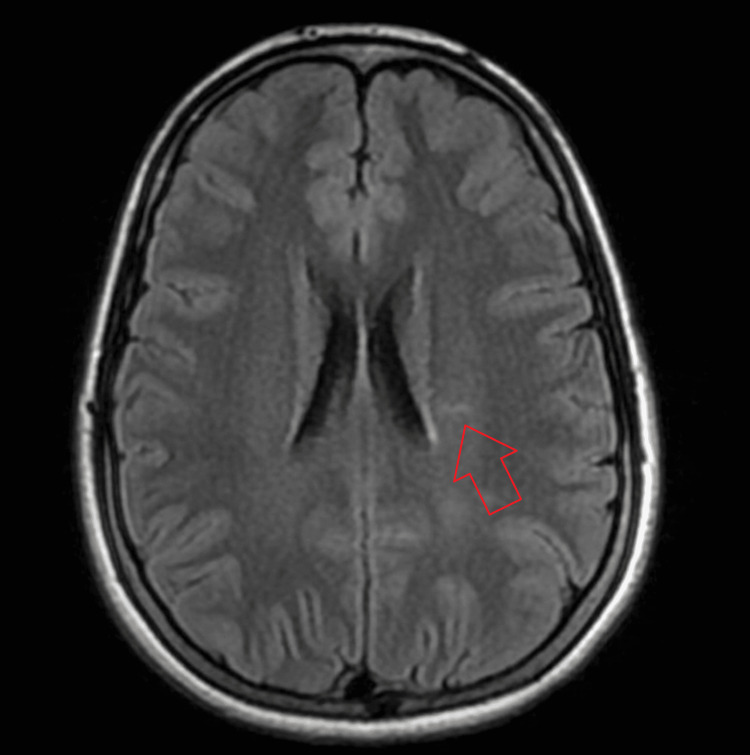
Cranial MRI findings (axial T2-FLAIR sequences) demonstrating a linear periventricular deep white matter hyperintensity perpendicular to the left lateral ventricle (indicated by the red arrow). This orientation is characteristic of "Dawson’s fingers," a radiographic hallmark of inflammatory demyelination resulting from perivenular inflammation. Its presence reinforces the clinical diagnosis of internuclear ophthalmoplegia as a demyelinating event despite the lack of visible lesions in the medial longitudinal fasciculus. FLAIR: Fluid-Attenuated Inversion Recovery
